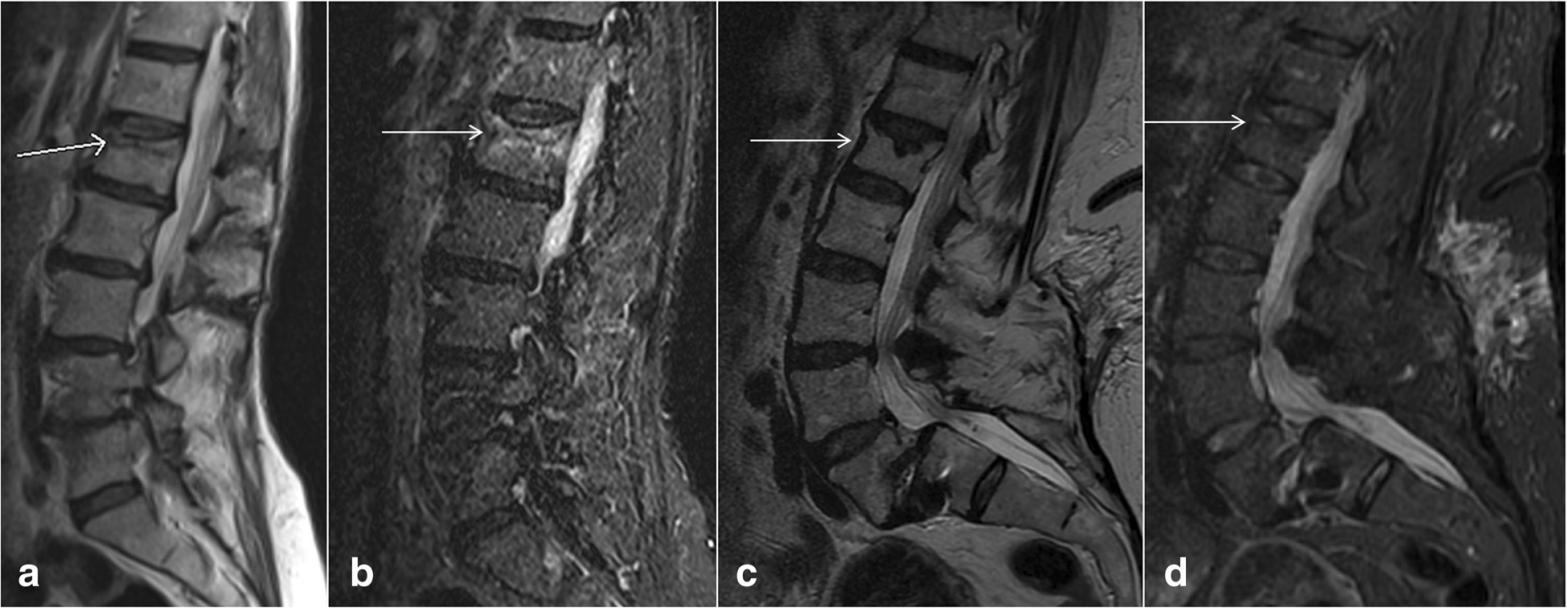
Sagittal T1 weighted image (a) and sagittal short tau inversion recovery (STIR) image (b) of a patient with an age-indeterminate fracture on CT showed bone marrow edema, suggestive of an acute vertebral body injury. Sagittal T1 weighted image (c) and sagittal STIR image (d) of a different patient with an age-indeterminate vertebral body fracture on CT showed no evidence of bone marrow edema, suggestive of a chronic injury
